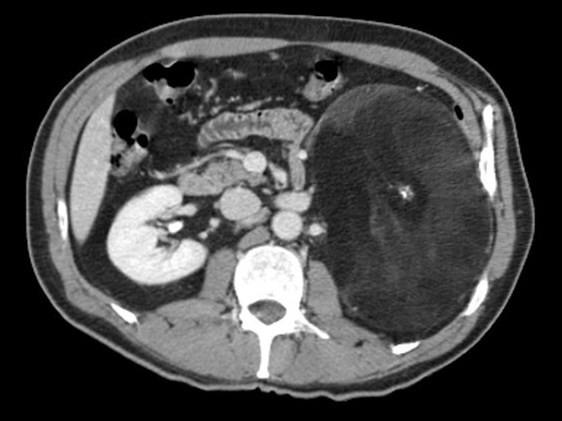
Myelolipoma identified by CT. Contrast-enhanced CT scan of the upper abdomen shows a large heterogeneous mass covering the upper left retroperitoneal space.
